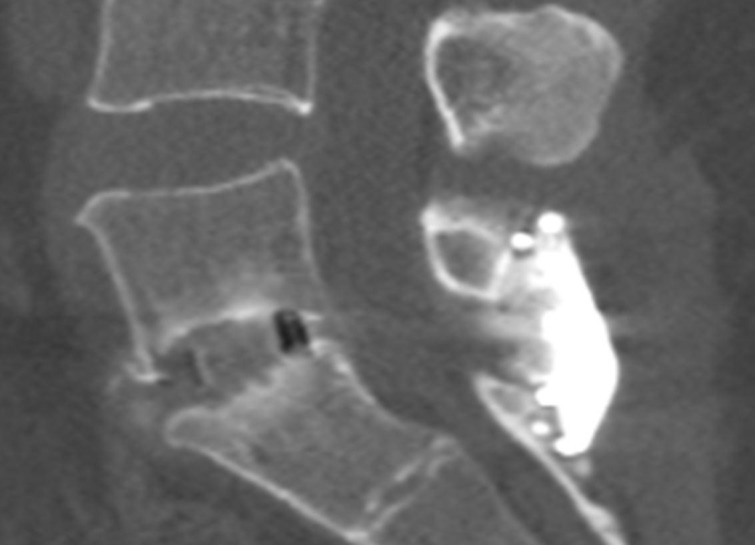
One-level transforaminal lumbar interbody fusion with interspinous process fixation; post-operative computed tomography scan (lateral view)
